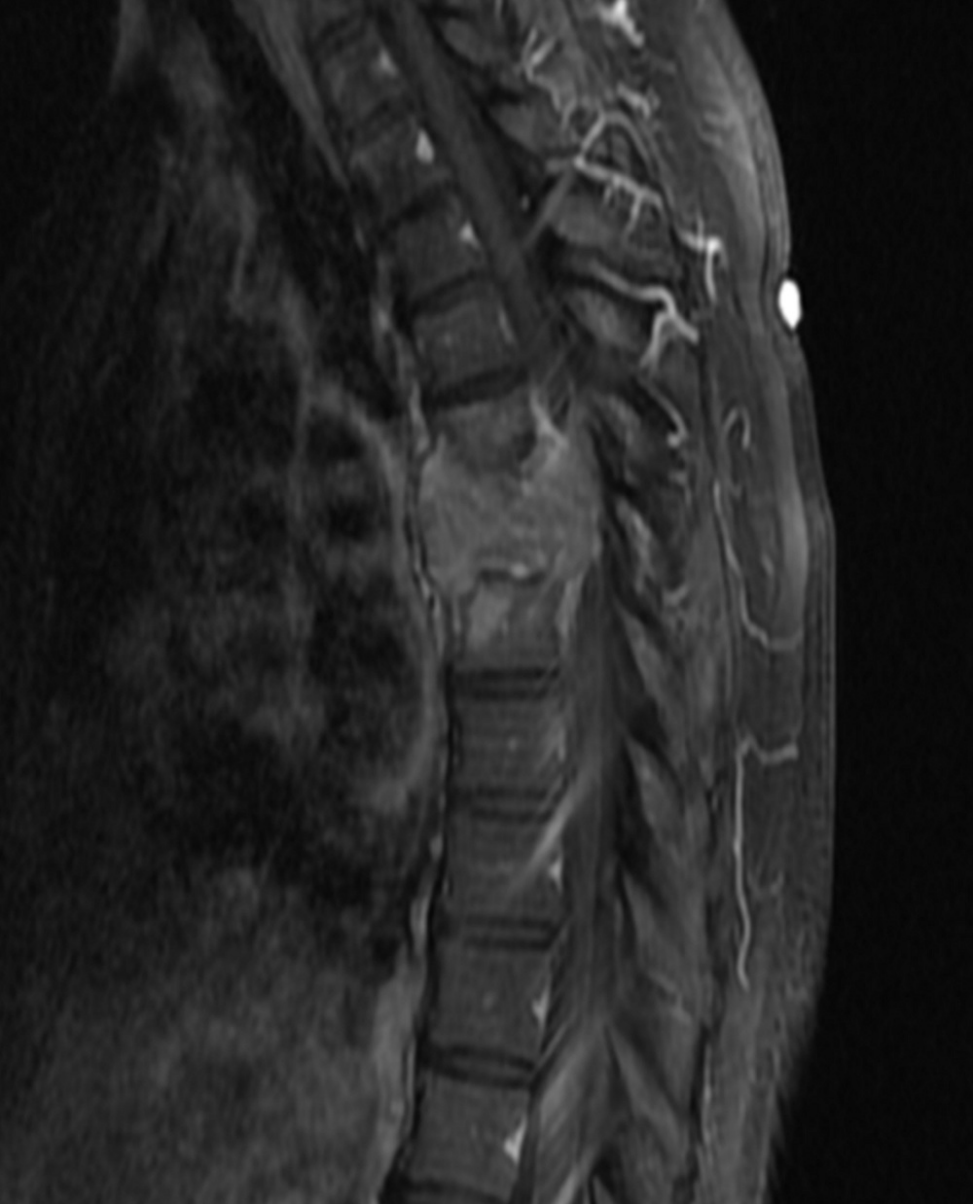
Sagittal T1 weighted MRI of thoracic spine with contrast Sagittal T1 weighted magnetic resonance imaging (MRI) of the thoracic spine with contrast demonstrating a homogeneously enhancing mass lesion in the T7 vertebral body invading superiorly and inferiorly into the intervertebral discs.
